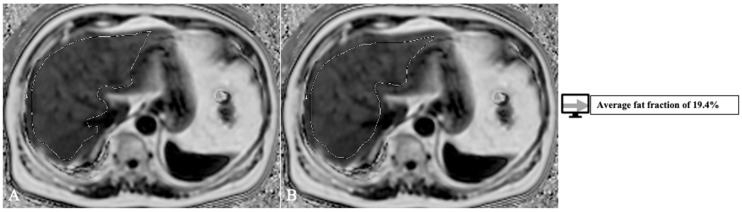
Exemplification of fat-fraction automatic quantification on MRI PDFF acquisitions with whole liver segmentation (1) at two different levels (A,B).
